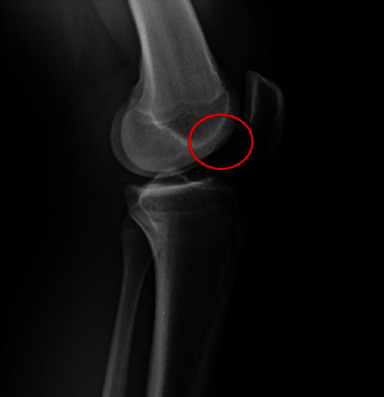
Lateral X-ray of a skeletally mature individual demonstrating a positive sulcus sign (red circle) suggestive of acute ACL injury.
